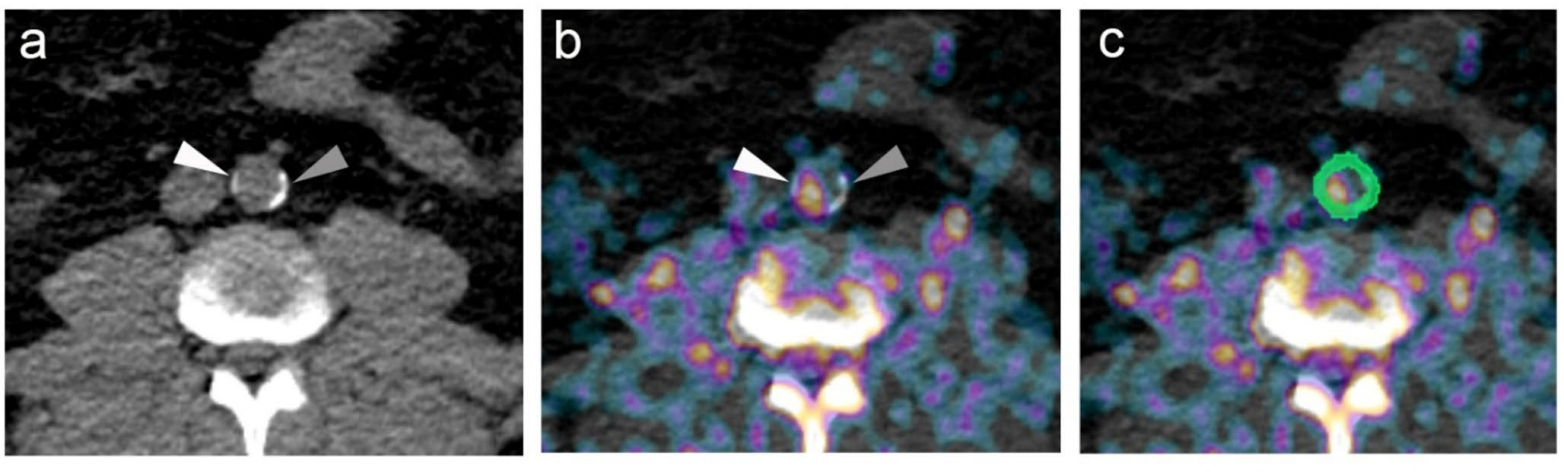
a Transversal CT scan of the abdominal aorta, where the white arrowhead indicates a smaller calcification, while the gray arrowhead points to a larger, denser calcification. b The same image overlaid with NaF-PET shows high NaF uptake in an area between the two CT-detectable calcifications, partially overlaying the smaller, less dense calcification (white arrowhead), while the larger, denser calcification (grey arrowhead) shows only a small amount of NaF uptake in the center where there is the least calcification on CT. c Segmentation of arterial wall performed by artificial intelligence shown in green color
